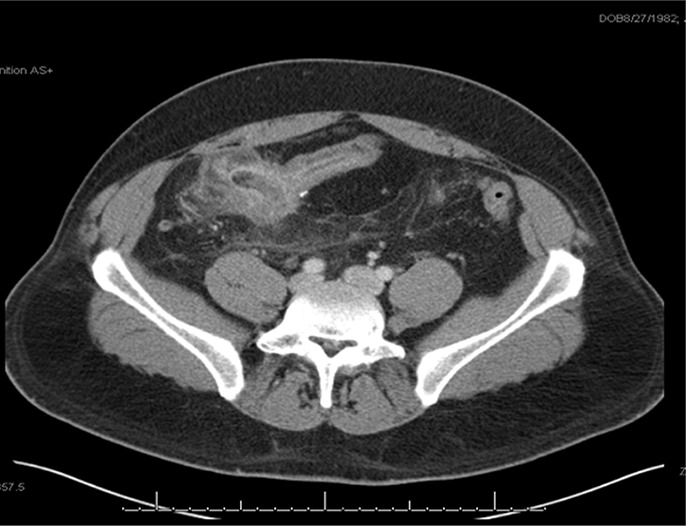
Abdominal computed tomography: thickened terminal ileum immediately adjacent to the sigmoid colon (shown by arrow) with no clear fat plane between bowel loops, which represents a fistula formation. Measurement 31.5 × 41.5 mm.
